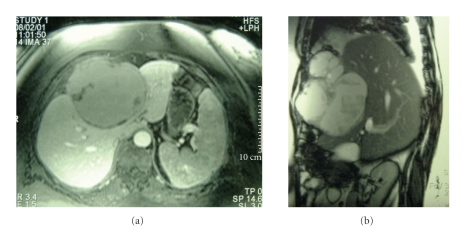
Magnetic resonance imaging (MRI) showing a cystic mass I segments IV and VIII, bulging into segments II and III and abutting the left and middle hepatic veins. The image also shows a soft tissue mass extending into the anterior abdominal wall.
